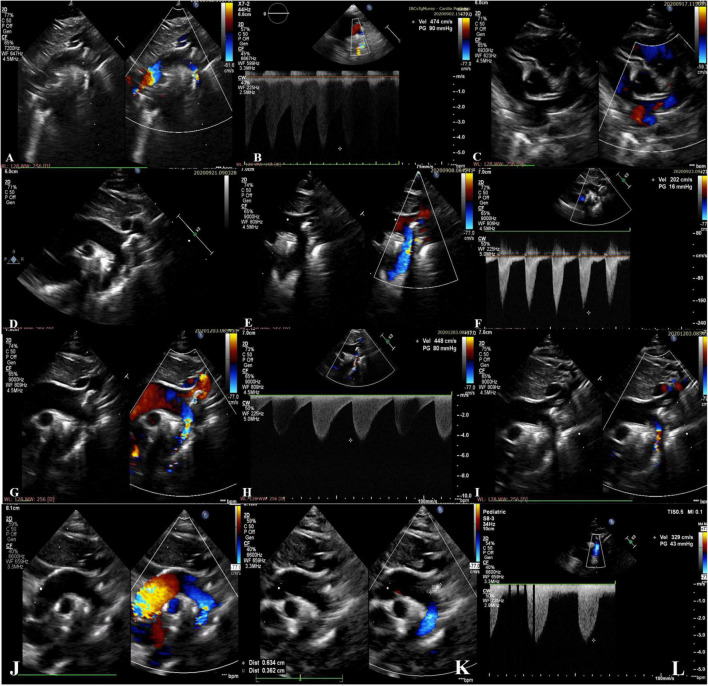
(A–C) Preoperative echocardiographic images from the neonatal period showing hyperechogenicity and severe narrowing of the aortic arch with a 2.5 mm diameter of the descending aorta lumen (gradient of 90mmHg) and normal coronary arteries; (D–F) postoperative echocardiographic images from the neonatal period showing the brightness and the abnormal echogenicity of the walls of the aortic arch and descending aorta and calcified residual masses at the level of the aortic arch extended to the brachiocephalic trunk, left common carotid artery, left subclavian artery, with laminar color Doppler flow in the descending aorta. Diameter of descending aorta lumen of 5.5 mm; (G–I) echocardiographic images at 3 months of age showing re-coarctation of the aorta with a significant gradient and the persistence of the aortic arch calcifications; (J–L) echocardiographic images at 18-months of age showing the regression of the aortic arch calcifications without obstruction, and mild left ventricular hypertrophy.
